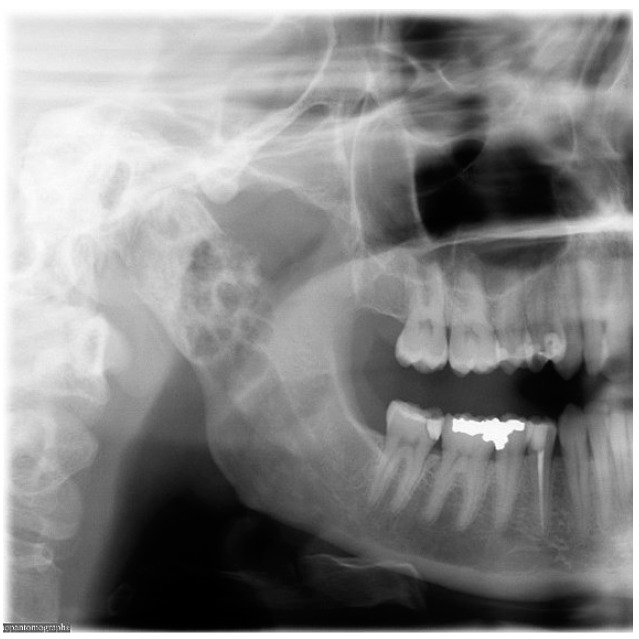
Orthopantomograph of the patient during first presentation showing a mixed radiopaque/radiolucent expansive lesion of the right mandibular condyle.
